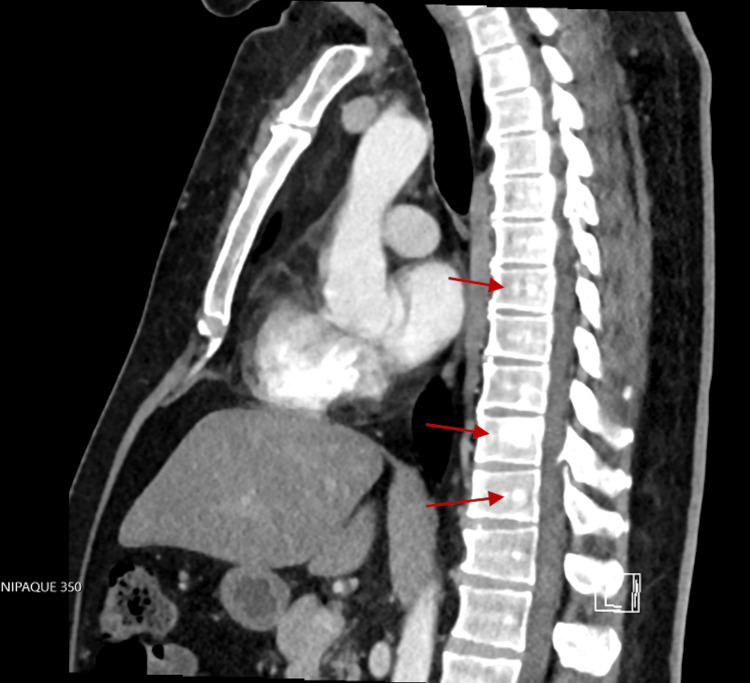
CT thorax showing sclerotic lesions in the spine
